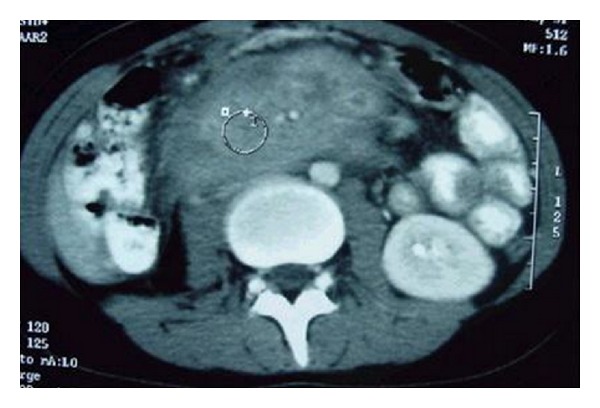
Abdominal computed tomography showing an 8 × 5 ×  7 cm heterogeneous lesion with indefinite borders invading to the proximal part of small bowel in retroperitoneal space.
